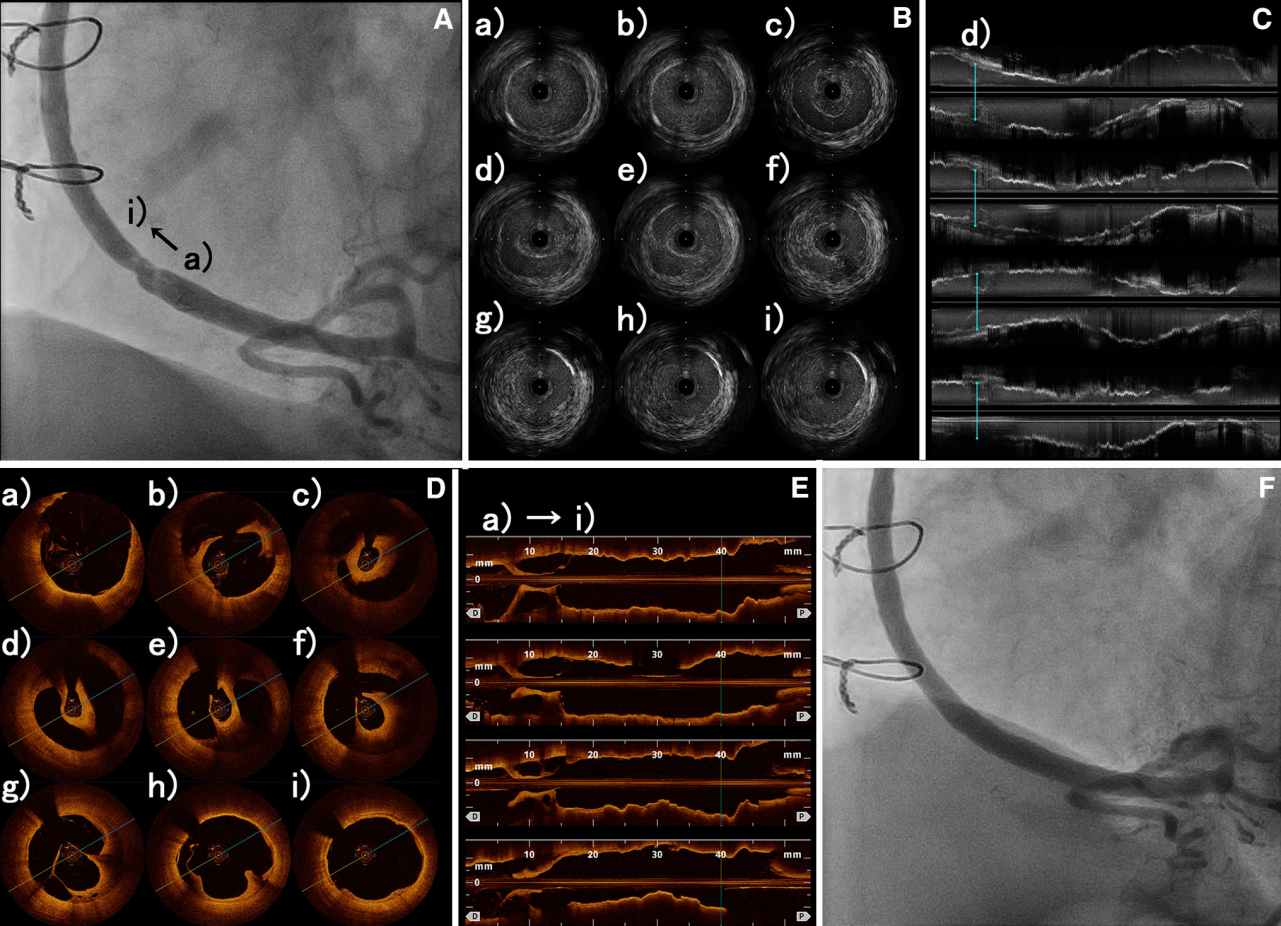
Comparison of intravascular ultrasonography (IVUS) and optical coherence tomography (OCT) images of the culprit lesion. A The saphenous vein graft (SVG) before percutaneous coronary intervention (PCI). Distal side of the culprit lesion was a, and proximal side was i. B, C IVUS, precise observation, and examination of the lesion in this case were insufficient. D Images visualized by short-axis OCT view. E Image visualized by long-axis OCT view had the appearance of a venous valve located in opposition to the blood flow. F The SVG after PCI
